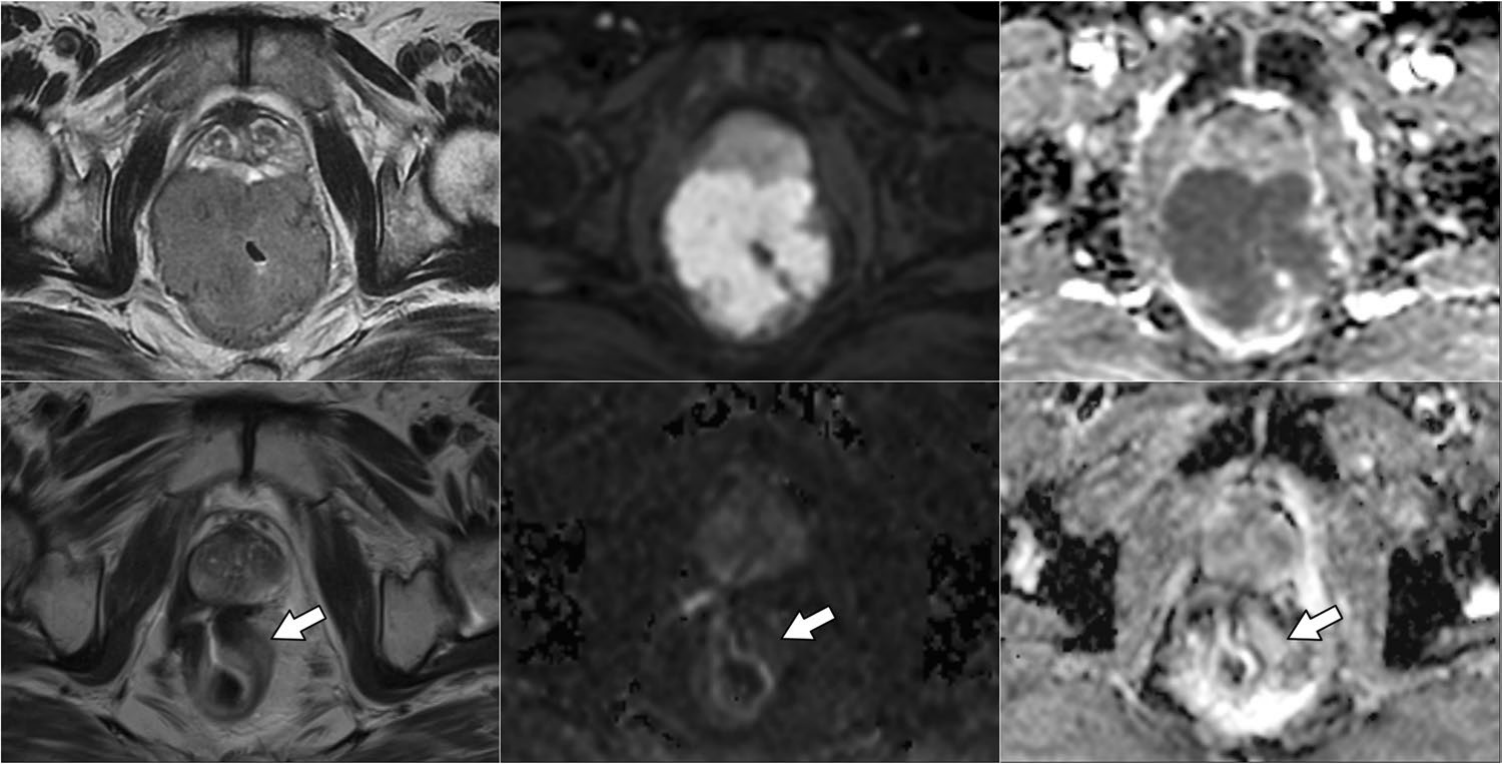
Images in a 57-year-old man with squamous anal carcinoma. T2-weighted MRI (right column), high *b*-value DWI (middle column) and DWI ADC map (left column). At baseline MRI, tumour staged as T4 invades the pelvic sidewall and prostate (upper row). After treatment (lower row), a region of indeterminate intermediate T2 signal in the lower rectal wall (TRG-3) does not correspond to restricted diffusion (arrows); linear diffusion restriction is spatially matched to anorectal mucosa (DWI-TRG-2)
